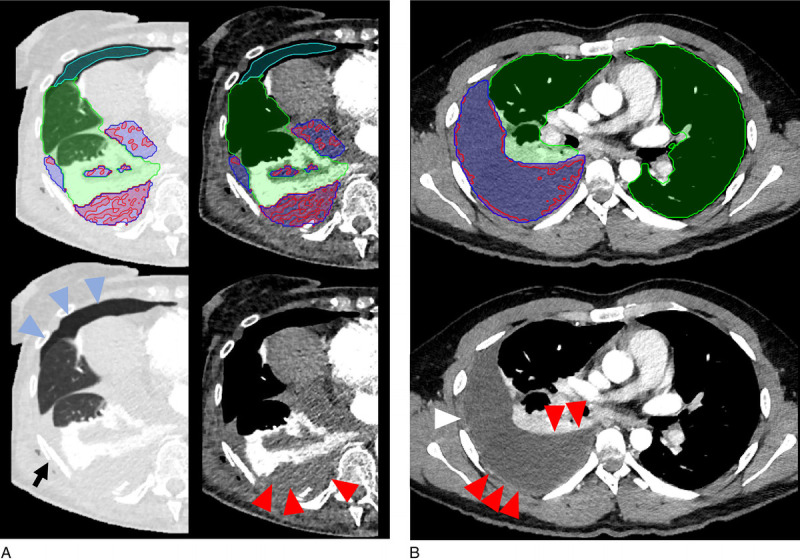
Classification results in 2 selected cases. Model predictions are shown at the top and original images at the bottom row. A, Posttraumatic chest CT of a patient with several rib fractures (black arrow), pneumothorax (blue arrowheads/light blue mask), and a complex effusion on the right (blue mask) with hyperdense fluid (red arrowheads and red mask) and with atelectasis of the lower lobe (green mask). B, Chest CT of a patient with empyema on the right side (blue) and no effusion on the left side. The hyperdense outer pleural space (HU >30 threshold, red mask) emphasizes the pleural thickening (red arrowheads).
